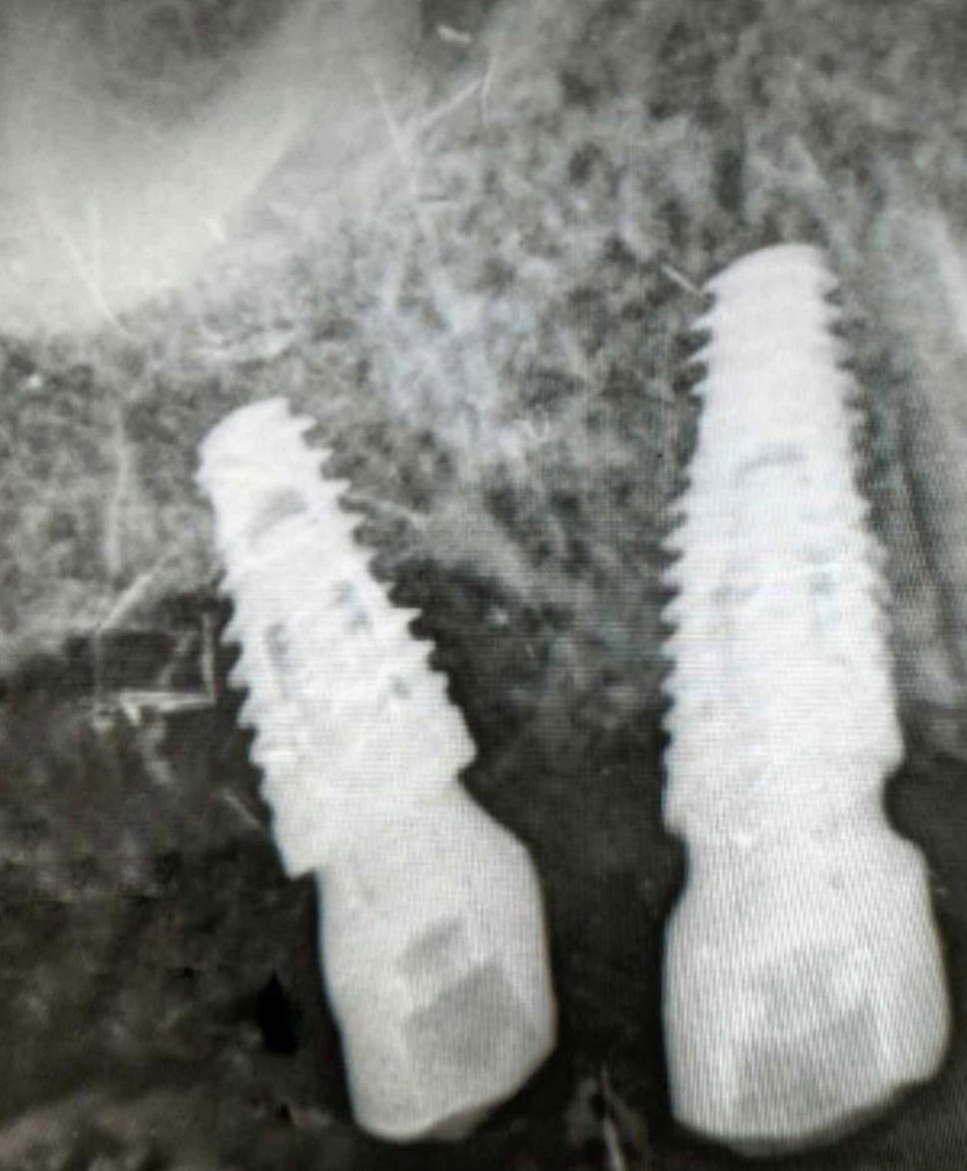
Radiographic assessment of peri-apical radiographic bone status
